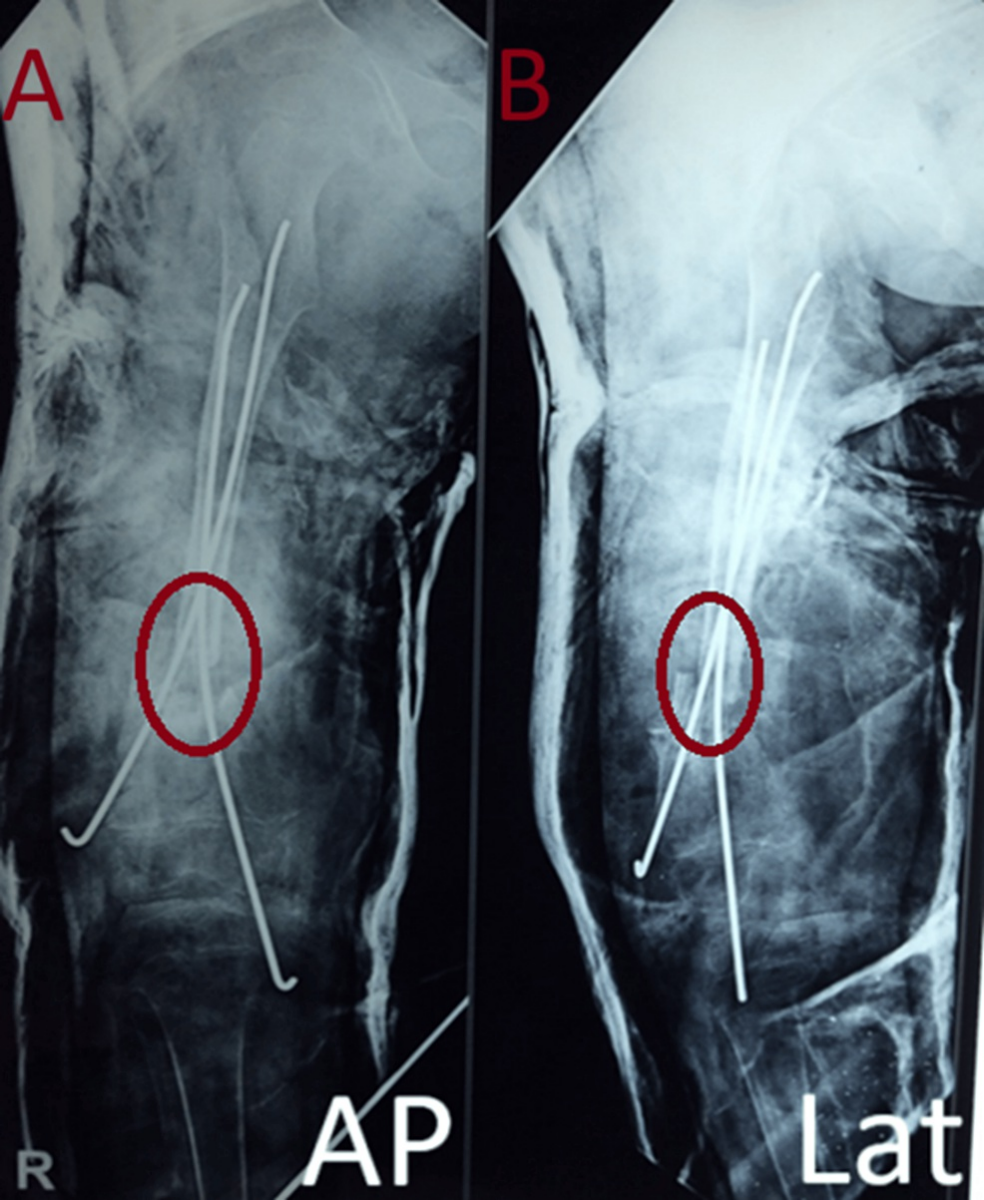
Immediate post-operation radiographs with right femur showing two K-wire fixations with fibular struct grafting at the distal one-third of right femur supplemented with one-and-a-half hip-spica A: AP view, B: Lateral view
